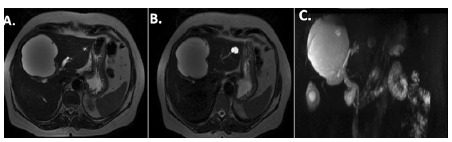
Pre-operative magnetic resonance image: A and B) axial image showing presence of central cystic lesion with thick wall and septum, in contact of hilar plate and placed in segments 4, 5, and 8; C) cholangio-resonance showing the contact to hilar plate and intra-hepatic dilation.
